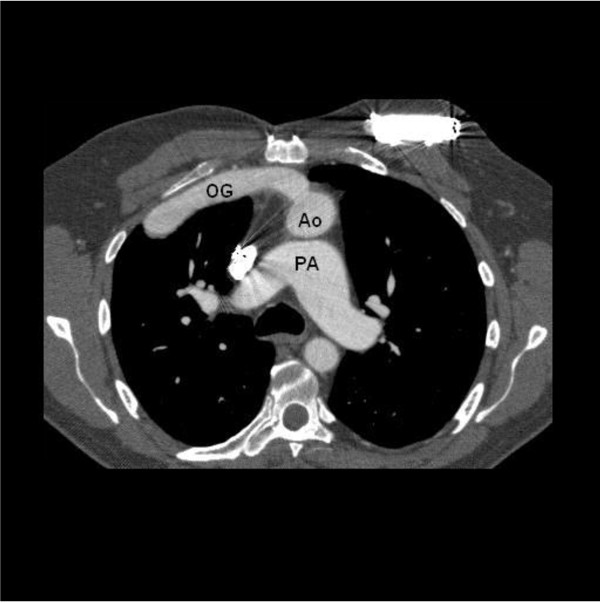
Contrast CT showing position of Outflow graft (OG) in relation to pulmonary artery (PA) and aorta (Ao).
